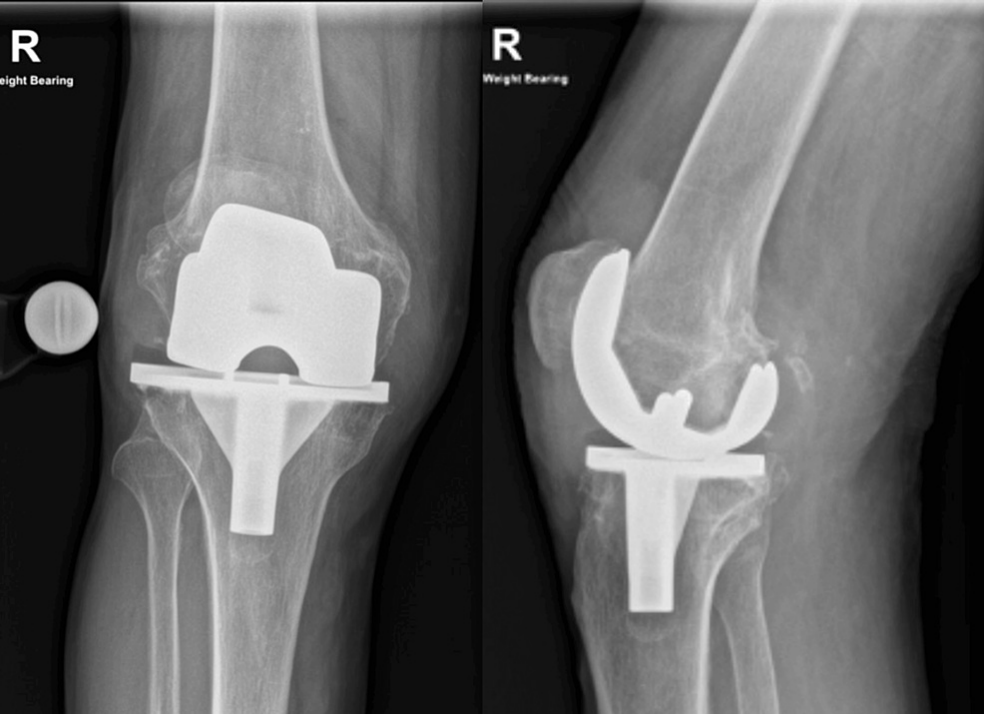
Anteroposterior and lateral X-rays of the right knee showing narrowing of the joint space.
